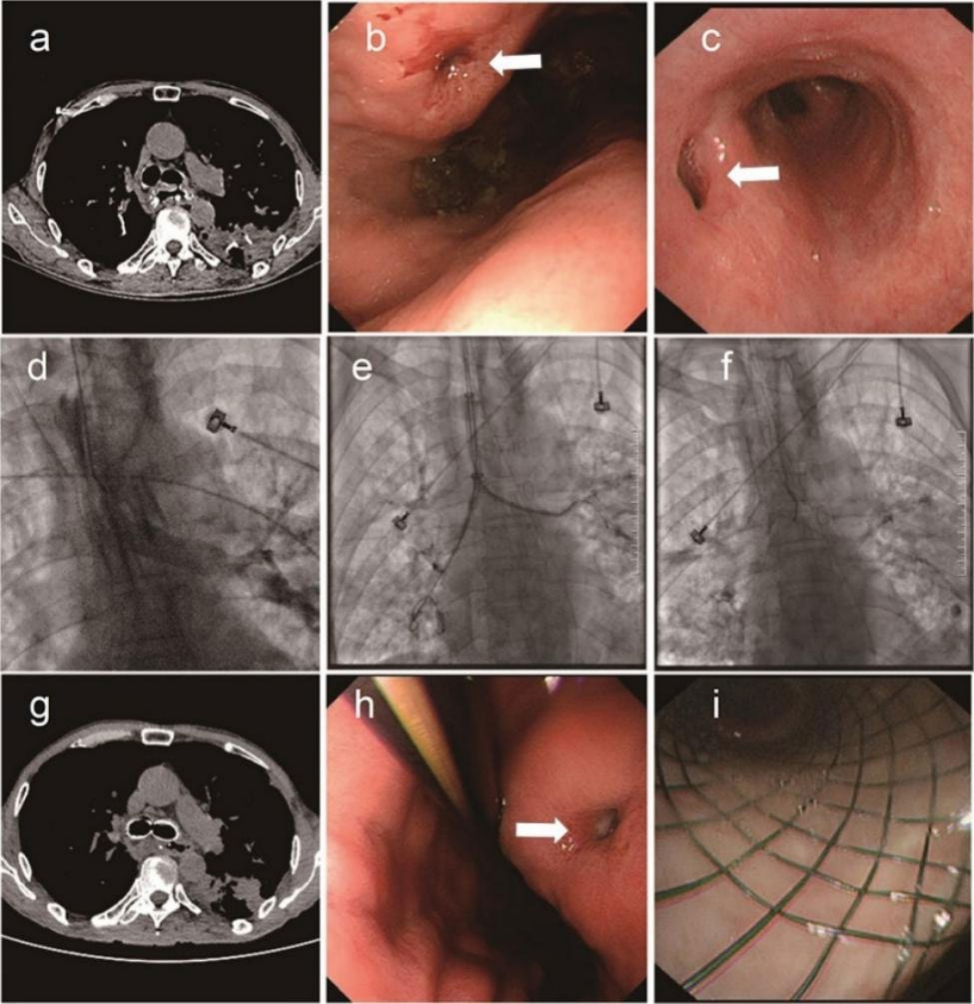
A GBF was treated by a covered Y-shaped self-expandable metallic stent. Representative images of GBF (arrow) from CT (a), gastroscopy (b), and bronchoscopy (c). Bronchogram was performed to find the GBF (d). Then, a covered Y-shaped self-expandable metallic stent was placed (e) and released (f). After stent placement, CT (g), gastroscopy (h), and bronchoscopy (i) were performed again to observe the GBF
